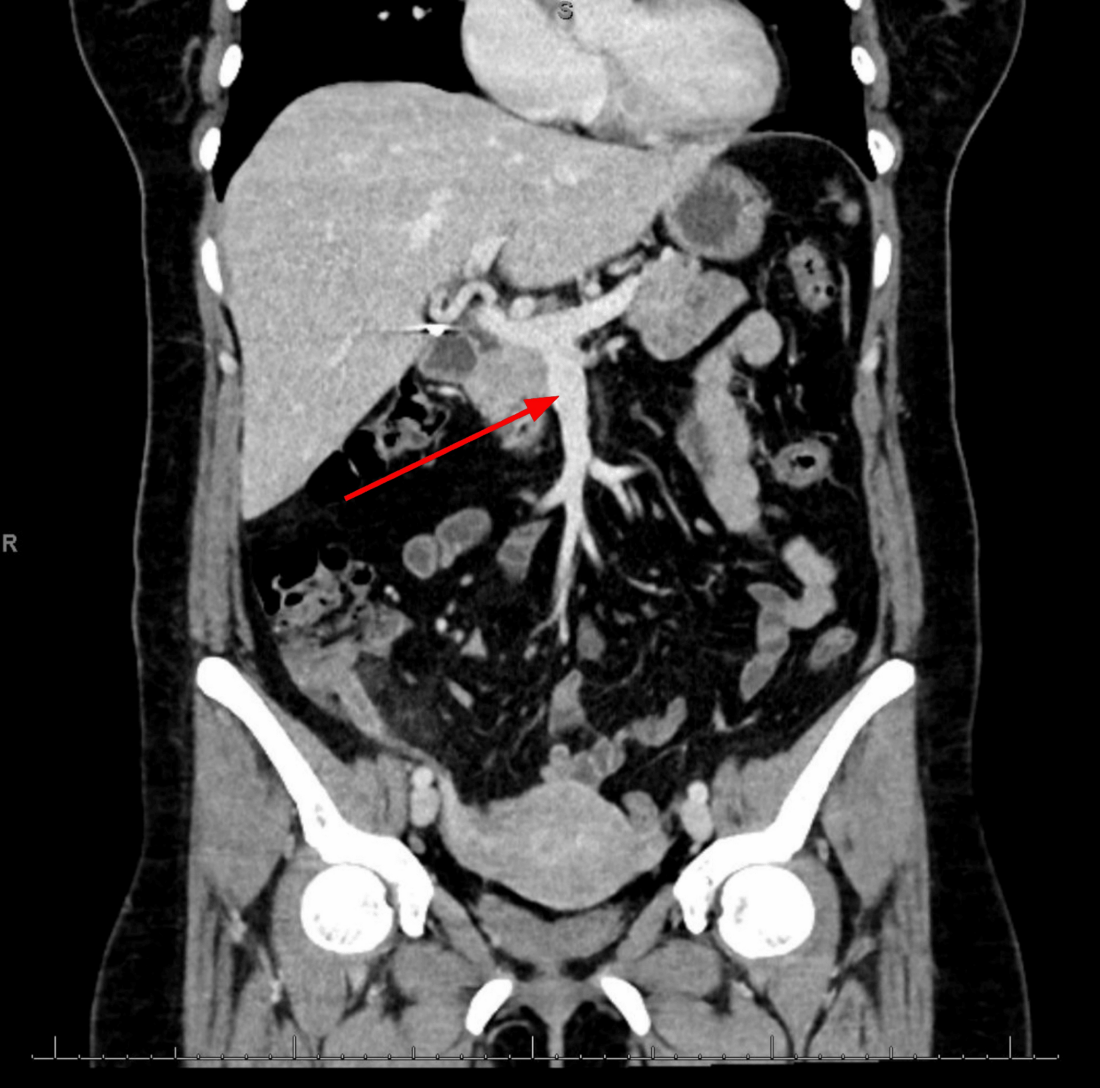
Coronal CT of the abdomen taken immediately before the patient's appendectomy, demonstrating no evidence of thrombus within the mesenteric veins (red arrow) CT: computed tomography
